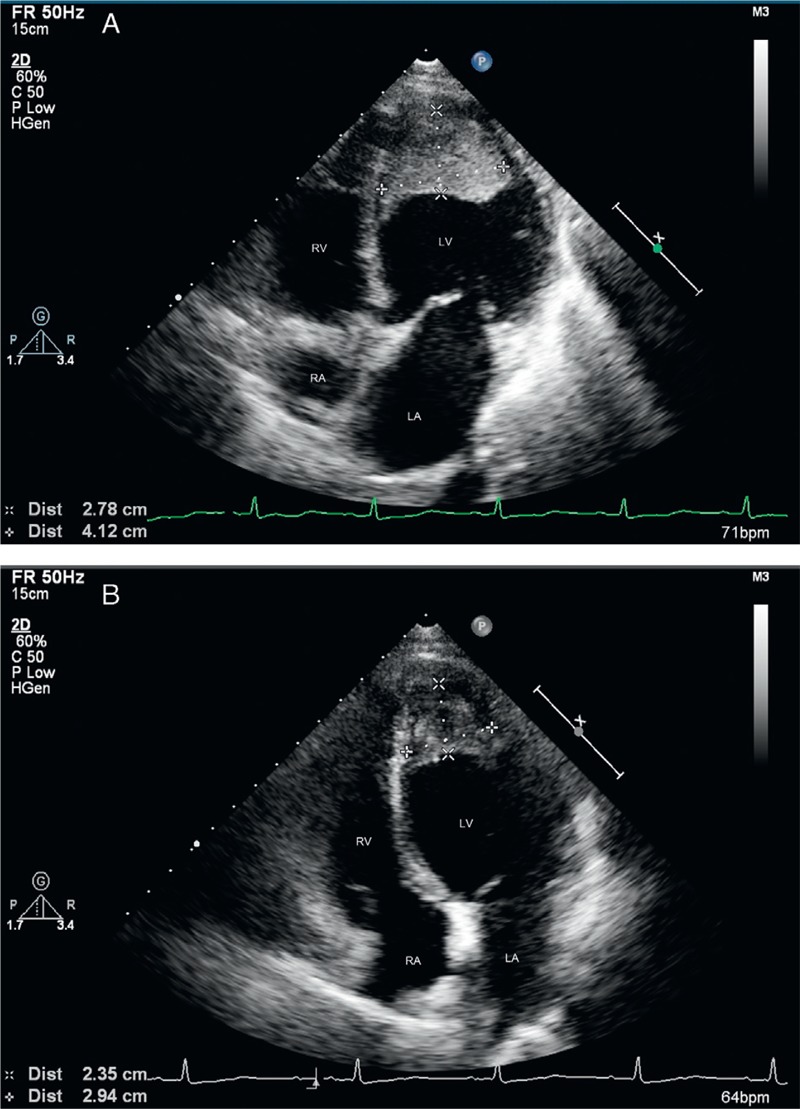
Images of 2D TTE examination of the first patient. (A) shows a half-moon-shaped heterogeneous echo of a thrombus measuring 41.2 × 27.8 mm at the LV apex. (B) shows that the size of the thrombus was decreased to 29.4 × 23.5 mm at 5 month follow-up. 2D = 2-dimensional, LV = left ventricular, TTE = transthoracic echocardiography.
